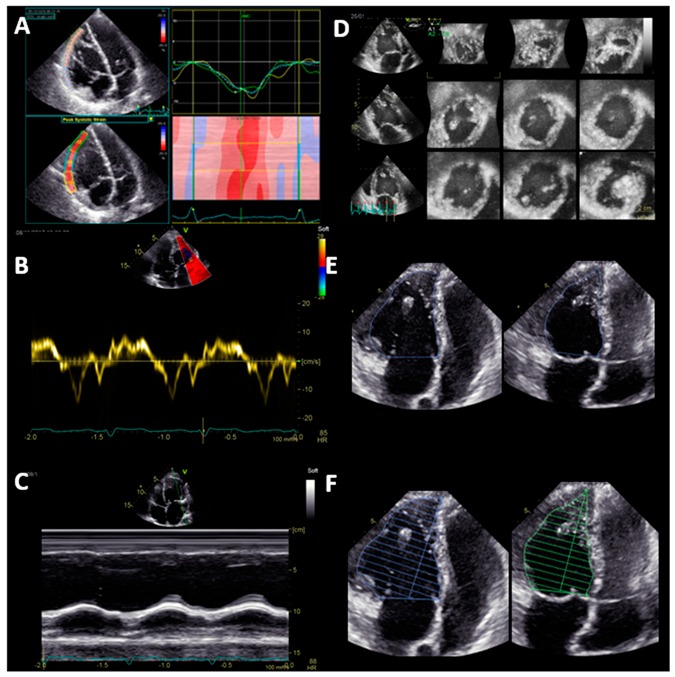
Systematic echocardiographic evaluation of systemic right ventricular function: Longitudinal function (Panel A-C) and volumetric function (Panel D-F). (A) Global longitudinal strain of the free lateral wall; (B) tissue Doppler velocity of the basal lateral wall S’; (C) tricuspid annular plane systolic excursion (TAPSE); (D) volumetric function assessed by 3D echocardiography; (E) fractional area change (FAC); (F) monoplane Simpson ejection fraction.
